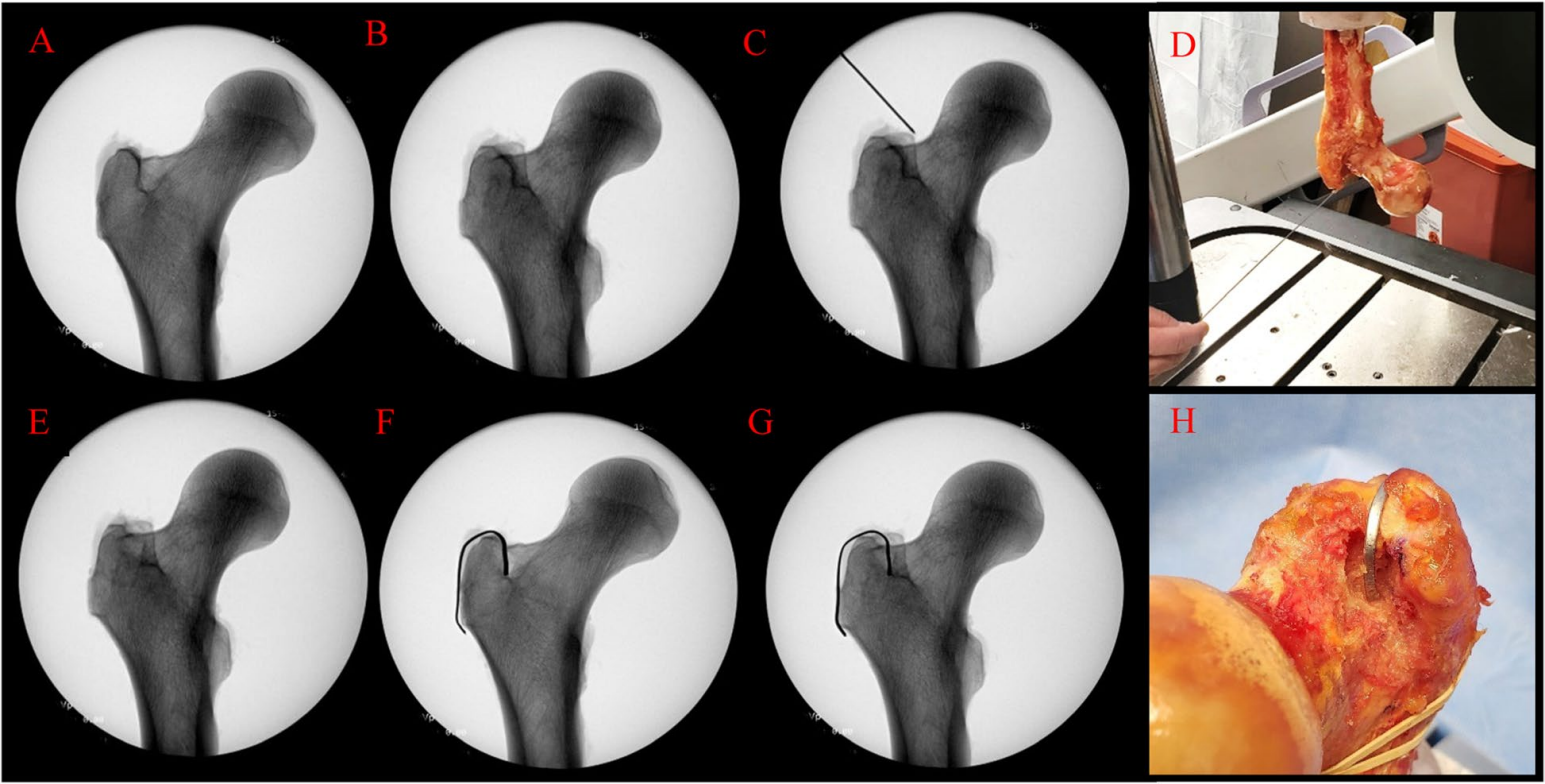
Radiographic and photographic images demonstrating (A) the presence of two superimposed radiographic lines on the medial greater trochanter at 0 degrees, (B) the presence of two radiographic lines on the medial greater trochanter at 30 degrees, (C) a K-wire in line with the posterior line radiographically, (D) the physical location of the K-wire as the radiograph in image c was taken, (E) the disappearance of the anterior radiographic line after bone has been removed at 30 degrees, (F) the reappearance of the anterior greater trochanteric line when the lead strip is placed in the center of the removed bone at 0 degrees, (G) the reappearance of the anterior greater trochanteric line when the lead strip is placed in the center of the removed bone at 30 degrees, (H) the physical location of the lead strip corresponding to the reappearance of the radiographic lines in images f and g
